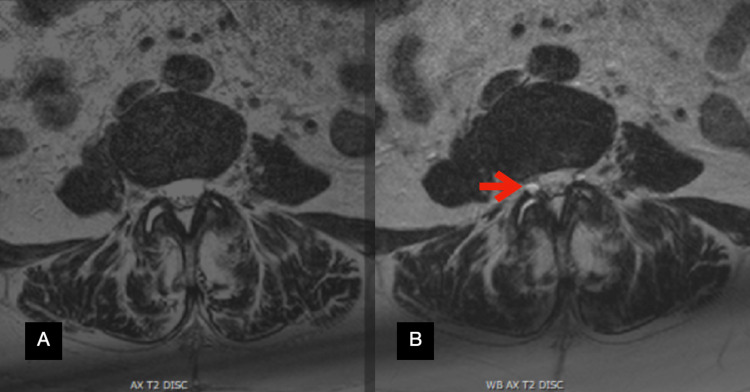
Axial T2-weighted MRIs at the L3-L4 facet joint without weight-bearing (A) and with weight-bearing (B) showing the development of a facet cyst (arrow).
